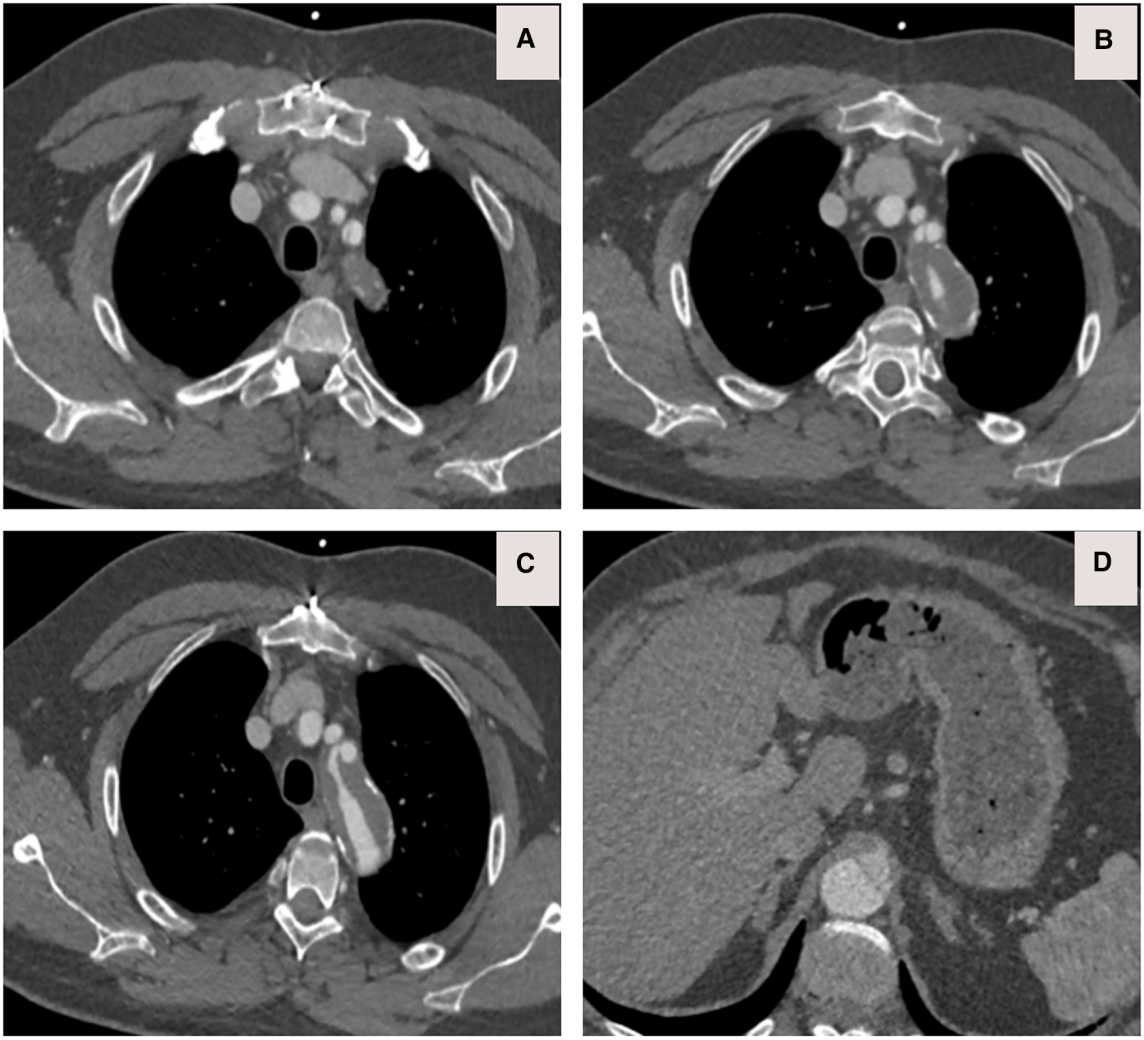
(A) CTA axial view depicts that the dissection extends to the left subclavian artery. (B,C) CTA axial views confirm the ominous sign of acute false lumen thrombosis that supplies visceral arteries. (D) CTA Axial view demonstrates that the celiac axis arises from a false lumen.
